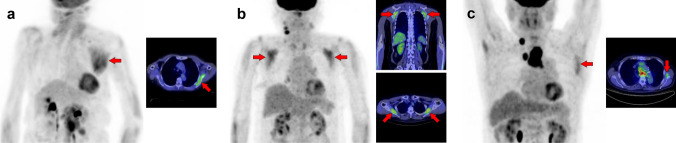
Subscapularis muscle.* We can observe a triangle-shaped distribution of subscapularis muscle (a, b, arrows) when [^18^F]FDG accumulates into the whole of the muscle. However, there are some cases where [^18^F]FDG uptake is found only in the lower parts, and it appears to be on the lateral side when the upper extremity is elevated (c, arrows)
